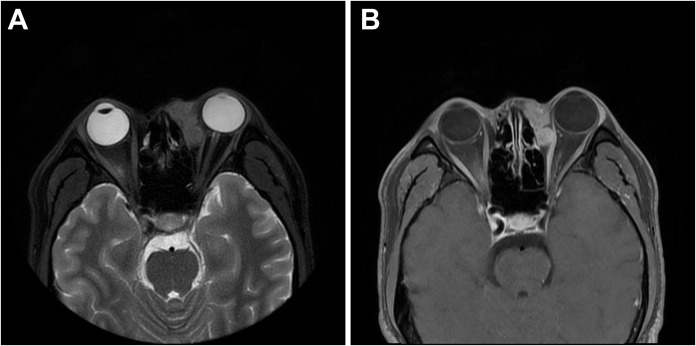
Imaging characteristics of the tumor on MRI. (A) Precontrast MRI scan; (B) Contrast-enhanced MRI scan.
